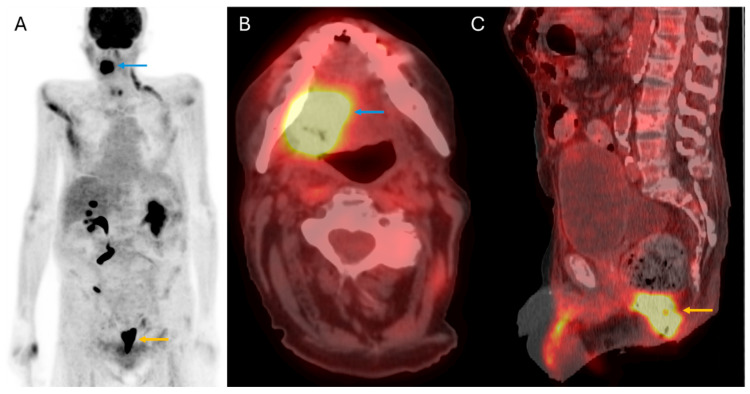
Synchronous primary malignancy detection: 70-year-old male with recurrent SCC of the oral cavity. Coronal MIP (A), axial fused PET/CT (B), and sagittal fused PET/CT (C) images demonstrate the primary recurrent malignancy in the oral cavity (blue arrows) and an incidentally detected hypermetabolic mass in the anal canal (yellow arrows), with a biopsy showing anal squamous cell carcinoma.
